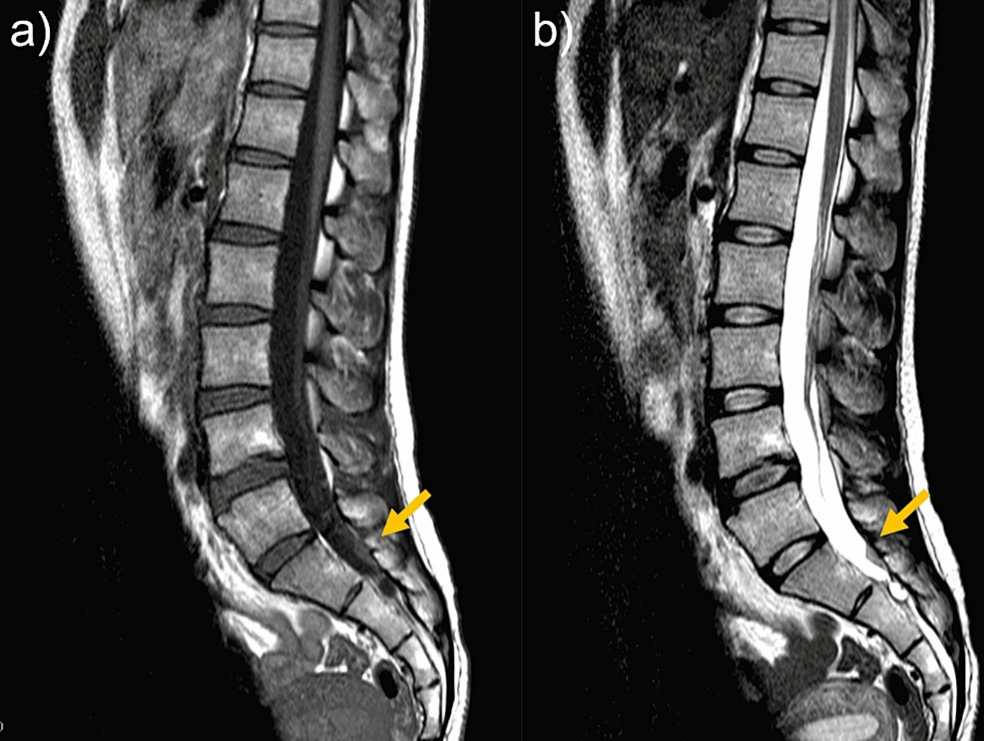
MRI at 1.5 months after onset (a) T1-weighted imaging (T1WI). (b) T2-weighted imaging (T2WI)
